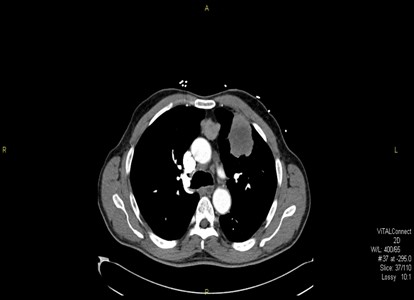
A CT scan with contrast of the chest showing large left upper lobe lung mass involving the pleural surface.
